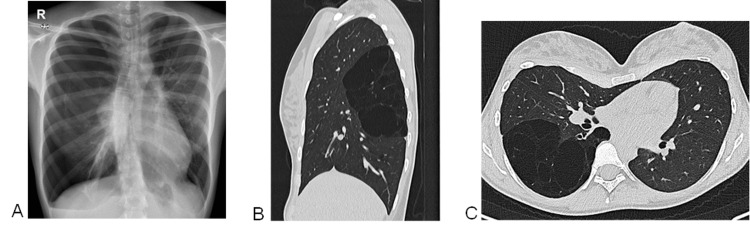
Imaging studies' findings (A) Chest radiography: large right-sided pneumothorax. (B) and (C): Chest CT - (B) sagittal and C) axial. Parenchymal rarefaction with panlobular emphysematous areas and air cysts, in the distribution of the apical segment of the right lower lobe. The right superior segmental lower lobe bronchus shows an apparent reduction in its caliber at its origin and downstream ectasia. Left deviation of mediastinum, conditioned by pectus excavatum.
